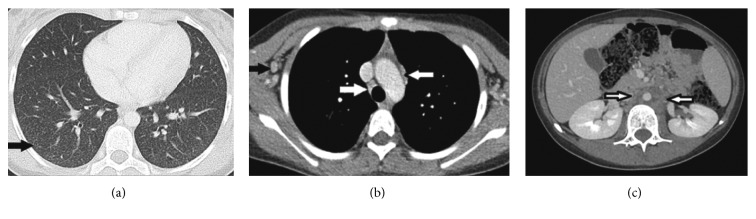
(a) Axial high-resolution chest computed tomography (CT) image through the lung bases shows multiple small (<3 mm) pulmonary nodules in a miliary pattern throughout both lungs with greatest concentration in the right lower lobe (black arrow). (b) Axial contrast-enhanced chest CT image through the superior mediastinum shows right axillary adenopathy (black arrow), right paratracheal adenopathy (white arrow), and prevascular space adenopathy (black-rimmed white arrow). (c) Axial contrast-enhanced CT image through the midabdomen shows low-density retroperitoneal adenopathy surrounding the descending thoracic aorta (black-rimmed white arrows).
